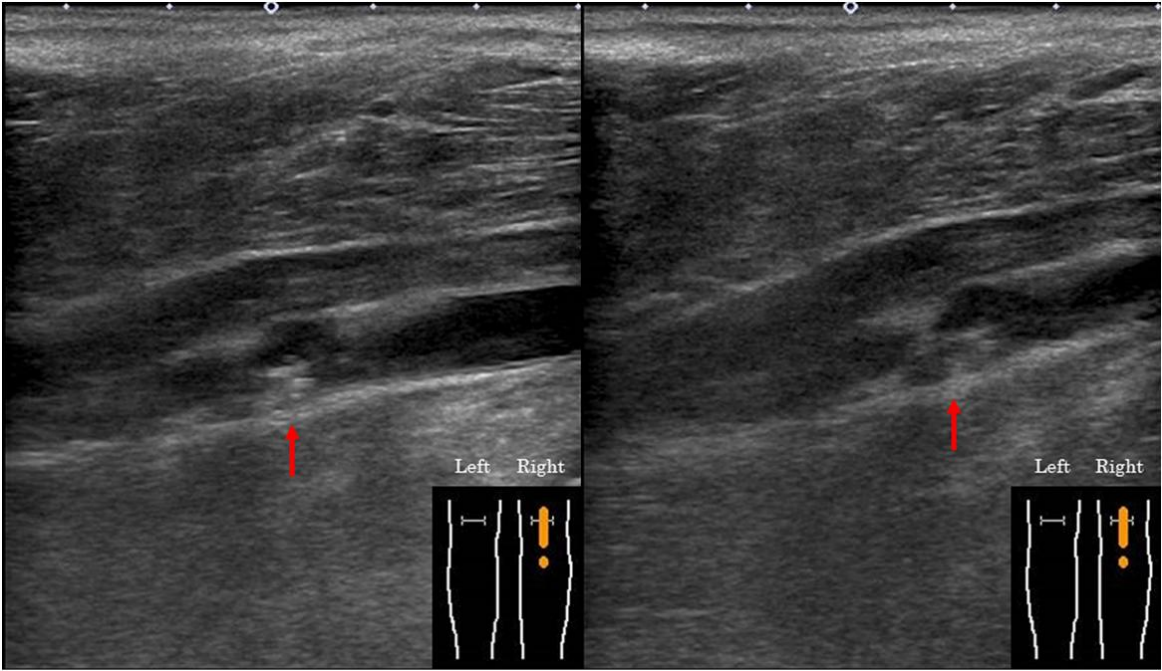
Lower extremity ultrasonography 6 months after discharge. Residual thrombus was observed (arrow).
